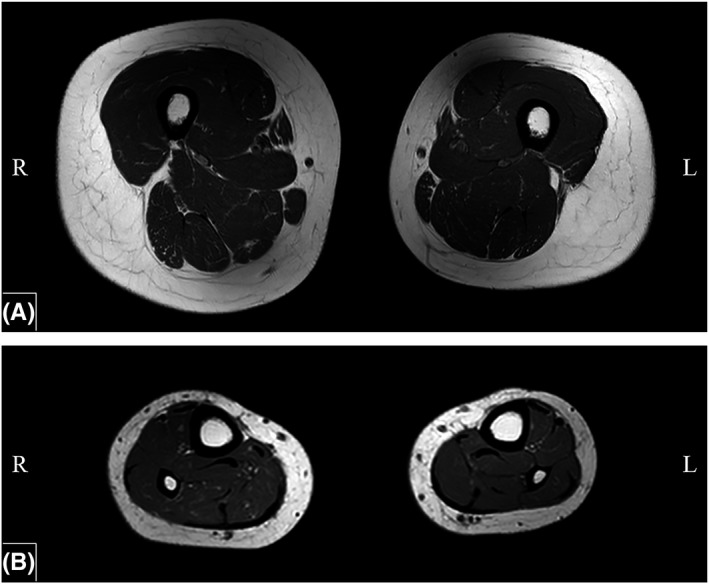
A, Bilateral Femur T1W Axial MRI without contrast. B, Bilateral Tibia/Fibula T1W Axial MRI without contrast
